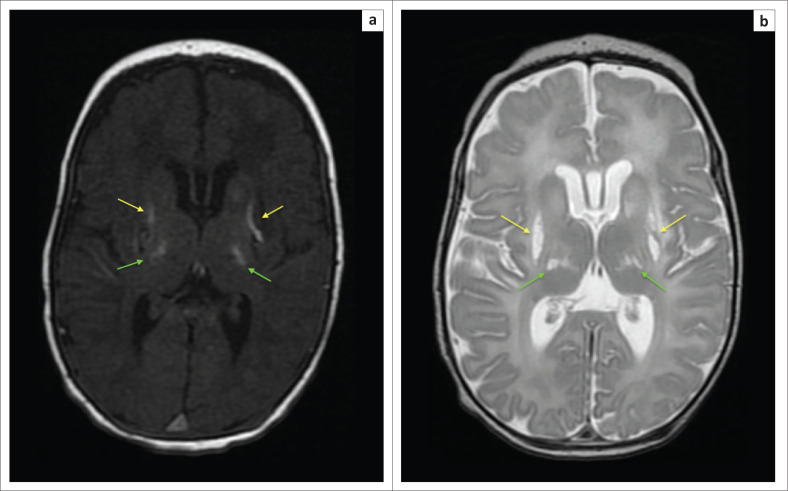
Twenty-seven-day-old male child delivered after abruptio placentae. Axial magnetic resonance imaging images at the level of the basal ganglia demonstrating bilateral fairly symmetrical dorsal putaminal (yellow arrows) and ventral thalamic (green arrows) hyperintensity because of T1 shortening (a) and corresponding T2-weighted hyperintensity and established atrophy of these structures (b). Note that the changes have evolved within the first month of life.
